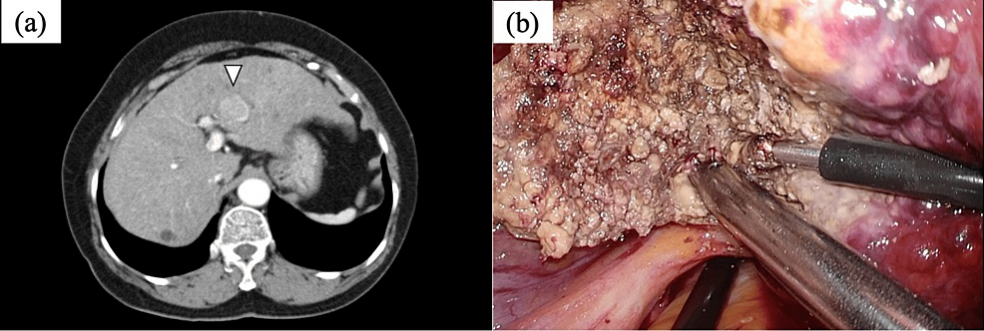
CT images and intraoperative findings of case 1 (a) A 3-cm-sized tumor in the S3 section of the liver was detected. (b) WJS was used to conduct laparoscopic S3 subsectionectomy. CT; computed tomography, WJS; water jet scalpel, S3; segment 3
